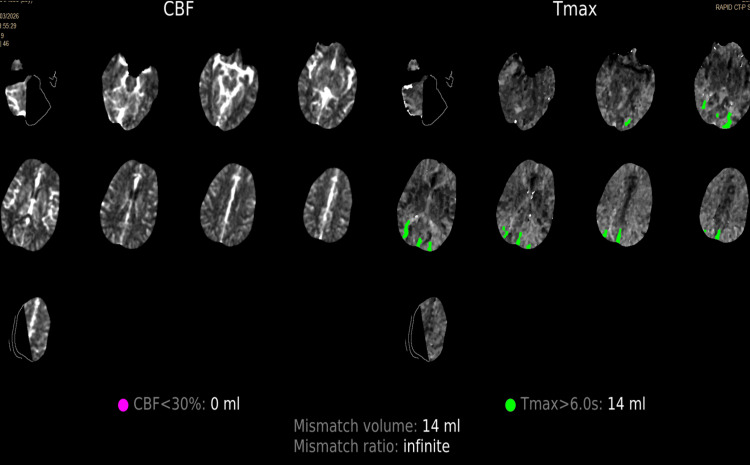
CT perfusion maps from the hyperacute stroke code study A non-specific apparent perfusion defect is visible and was reported by radiology as an artifact; the green Tmax signal corresponds to the motion-related artifact.
